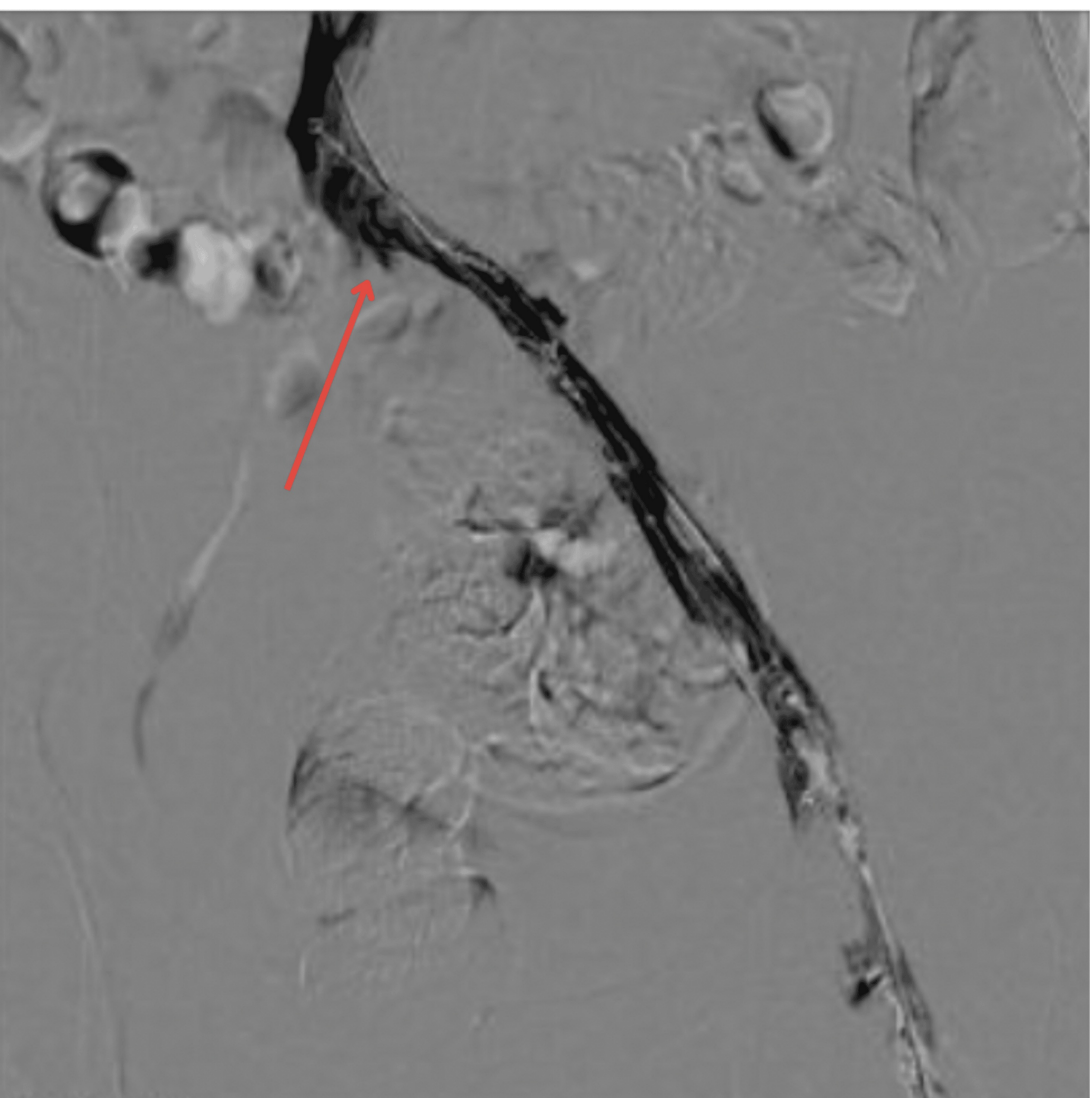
Flow restored post angioplasty but IVUS assessment confirmed persistent stenosis at the distal left common iliac vein (red arrow) IVUS: intravascular ultrasound
